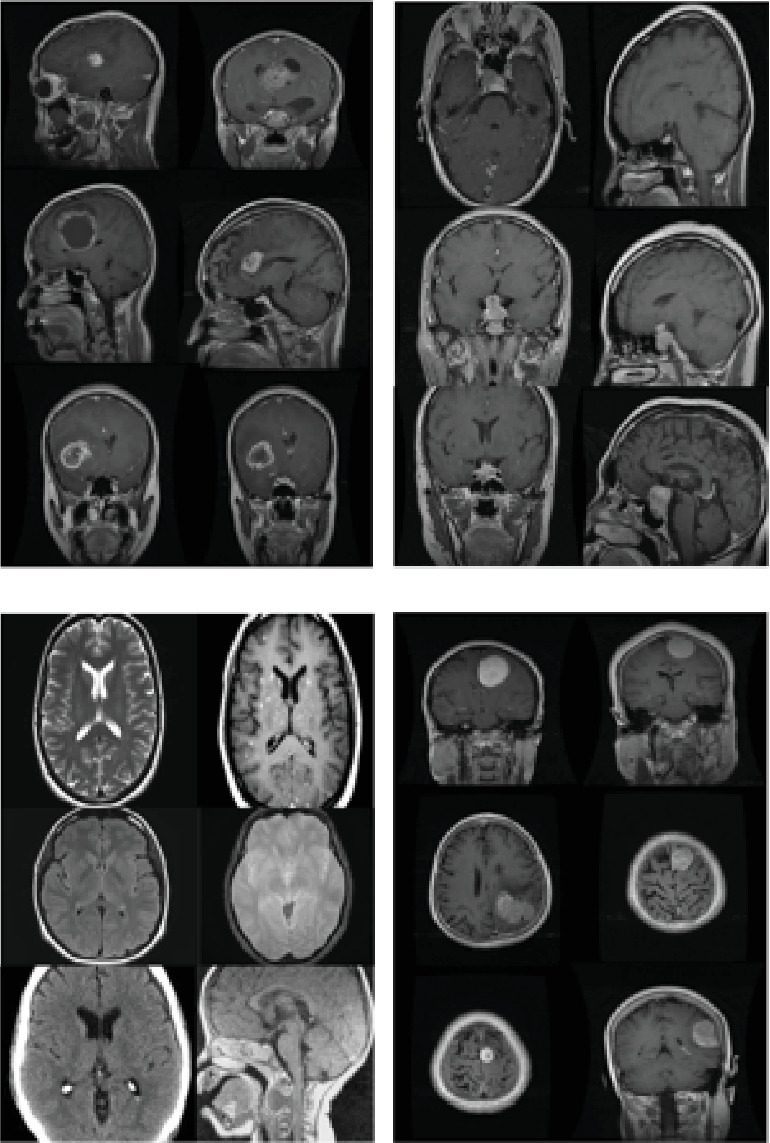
The brain tumours. MR images.
